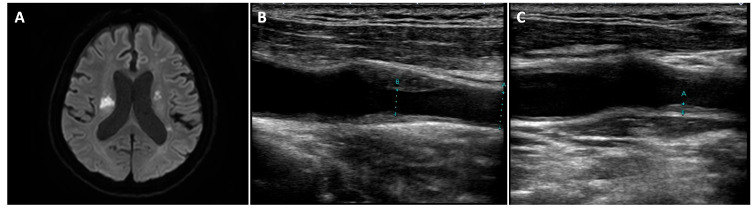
Diffusion-Weighted Magnetic Resonance Imaging (DW-MRI) of the brain and echocardiogram of the left and right carotid arteries. (A) Multiple infarcts in the left middle cerebral artery territory, right corona radiata, and right internal capsule shown in DW-MRI; (B,C) stenosis and plaque showed in the right (B) and left (C) carotid arteries.
